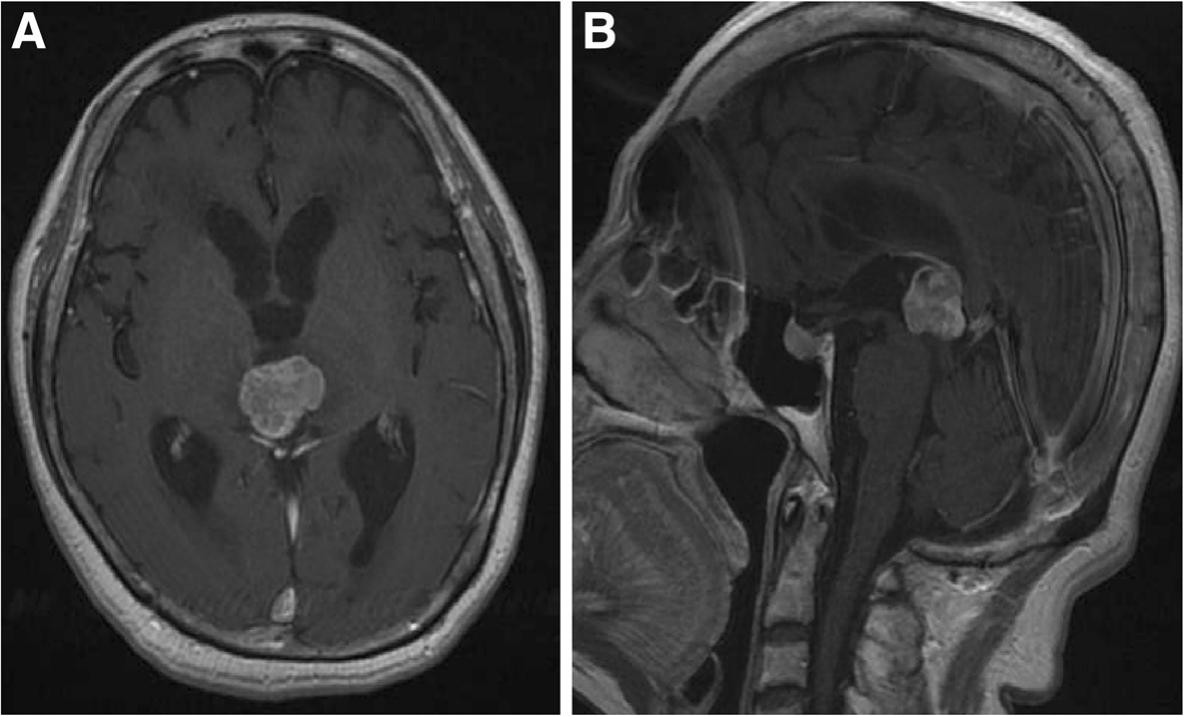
Magnetic resonance imaging scan of the brain at diagnosis. Magnetic resonance imaging with gadolinium injection showing a 25-mm pineal region tumor with heterogeneous enhancement associated with hydrocephalus on the T1-weighted axial image (A), and sagittal image (B).
